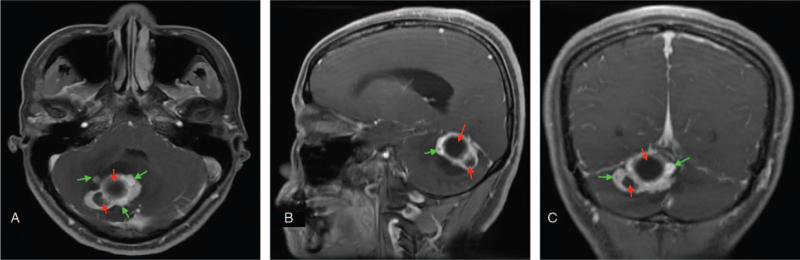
(A–C) Preoperative MRIs showing the two cystic lesions in the cerebellum with irregular ring enhanced cyst walls composed of smaller nodular parts. A = axial, B = sagittal, and C = coronary Red arrow = cystic lesion with ring-enhanced walls. Green arrows indicate tumor nodules. MRI = magnetic resonance imaging.
